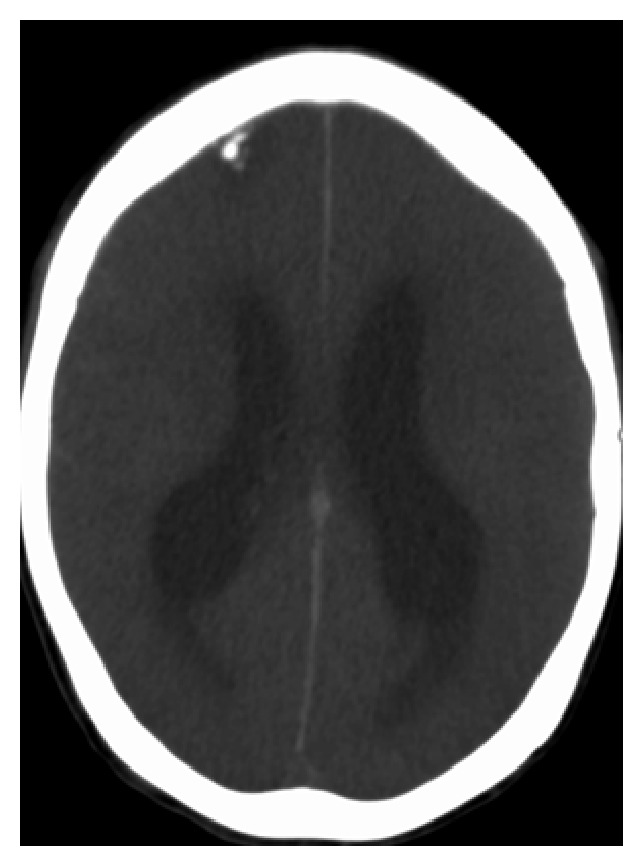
Head CT showing diffuse cerebral edema with ventriculomegaly.
